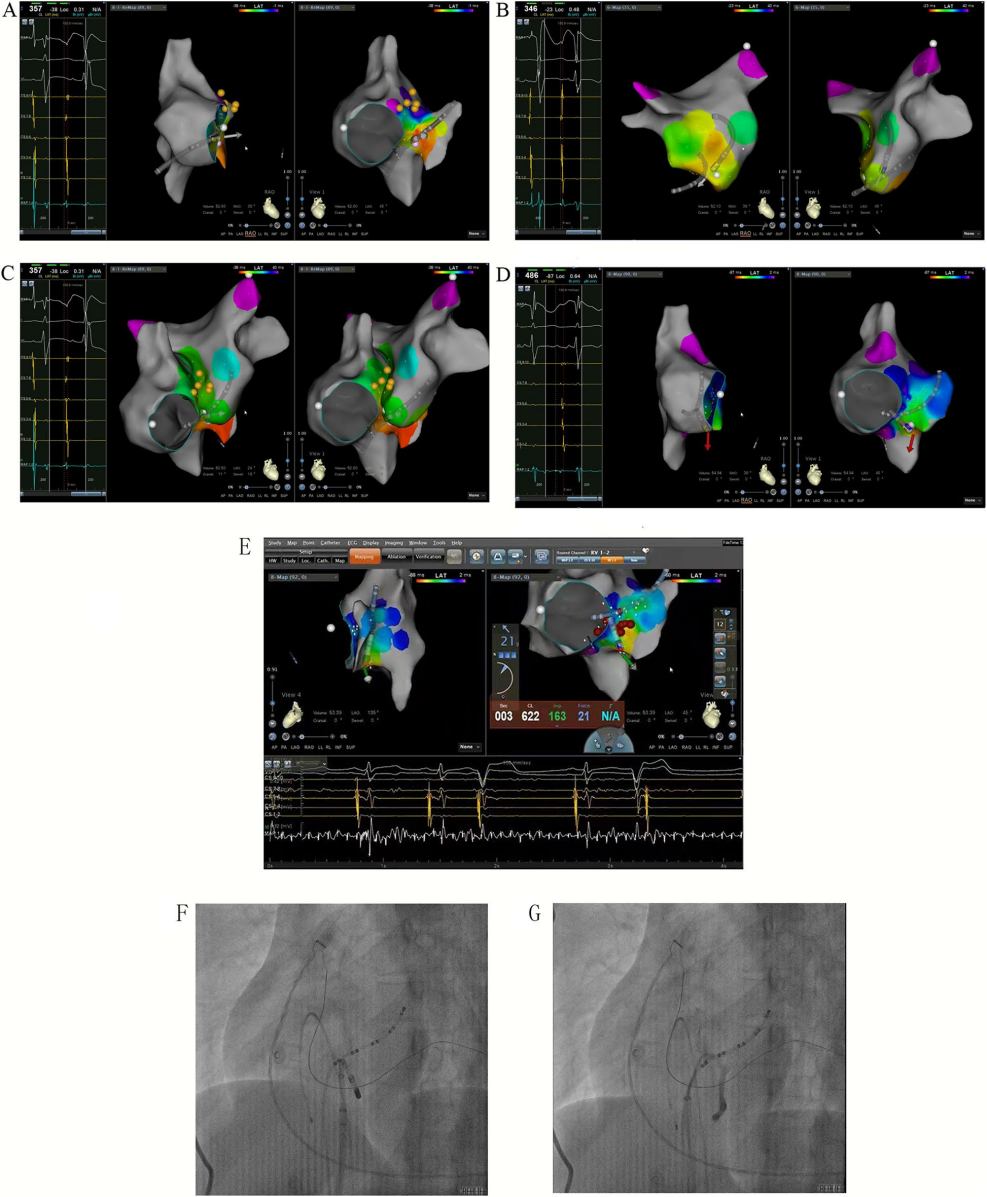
Three-dimensional (3D) activation map. A A ThermoCool SmartTouch catheter was used for the right atrium mapping, revealing 5–6 poles of the decapolar catheter as the earliest activation. B and C Mapping of the left atrium found no earlier atrial activity in the left posterior septum. D The earliest activation was near the site of the proximal middle cardiac vein (pMCV). There were low-amplitude, fractionated electrograms at the earliest activation site. E Three-dimensional image of RF ablation. F X-ray image of RF ablation (LAO fluoroscopic view). G Angiography through the multifunctional catheter confirmed that this successful ablation site was in the proximal MCV (LAO fluoroscopic view)
